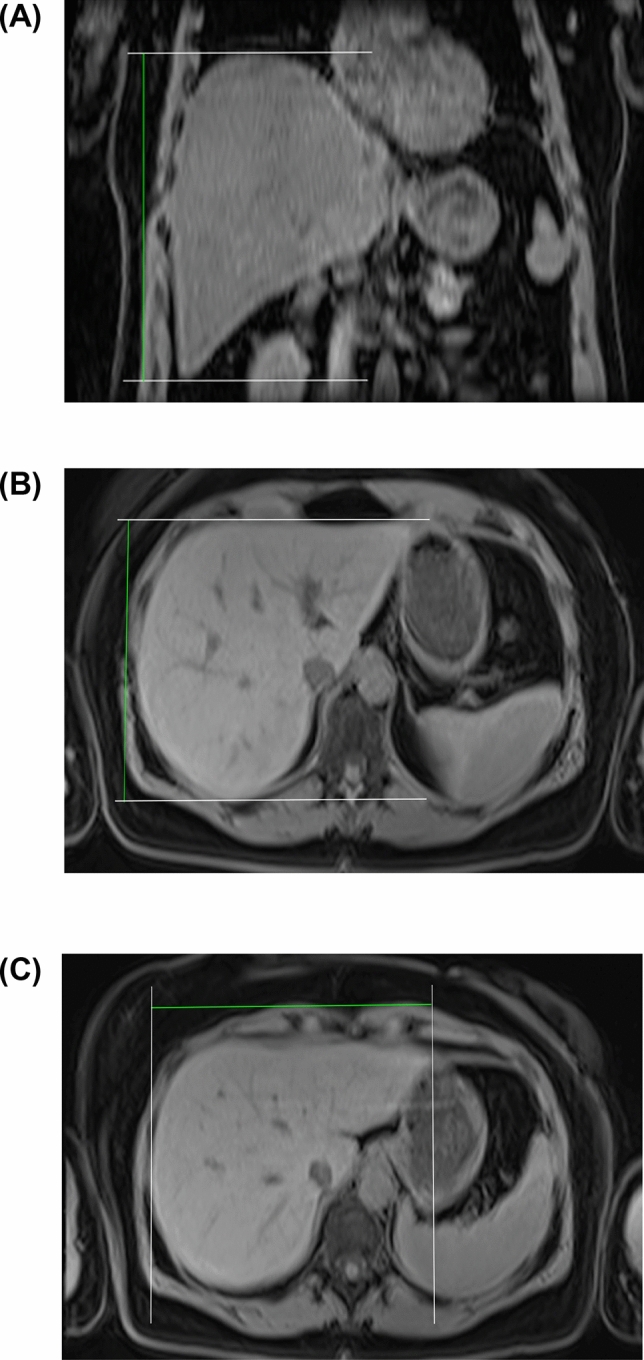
Sample measurement of diameters based on the MRI sectional images. Measurement of the maximum liver expansion in CC (A), AP (B) and ML (C) direction.
